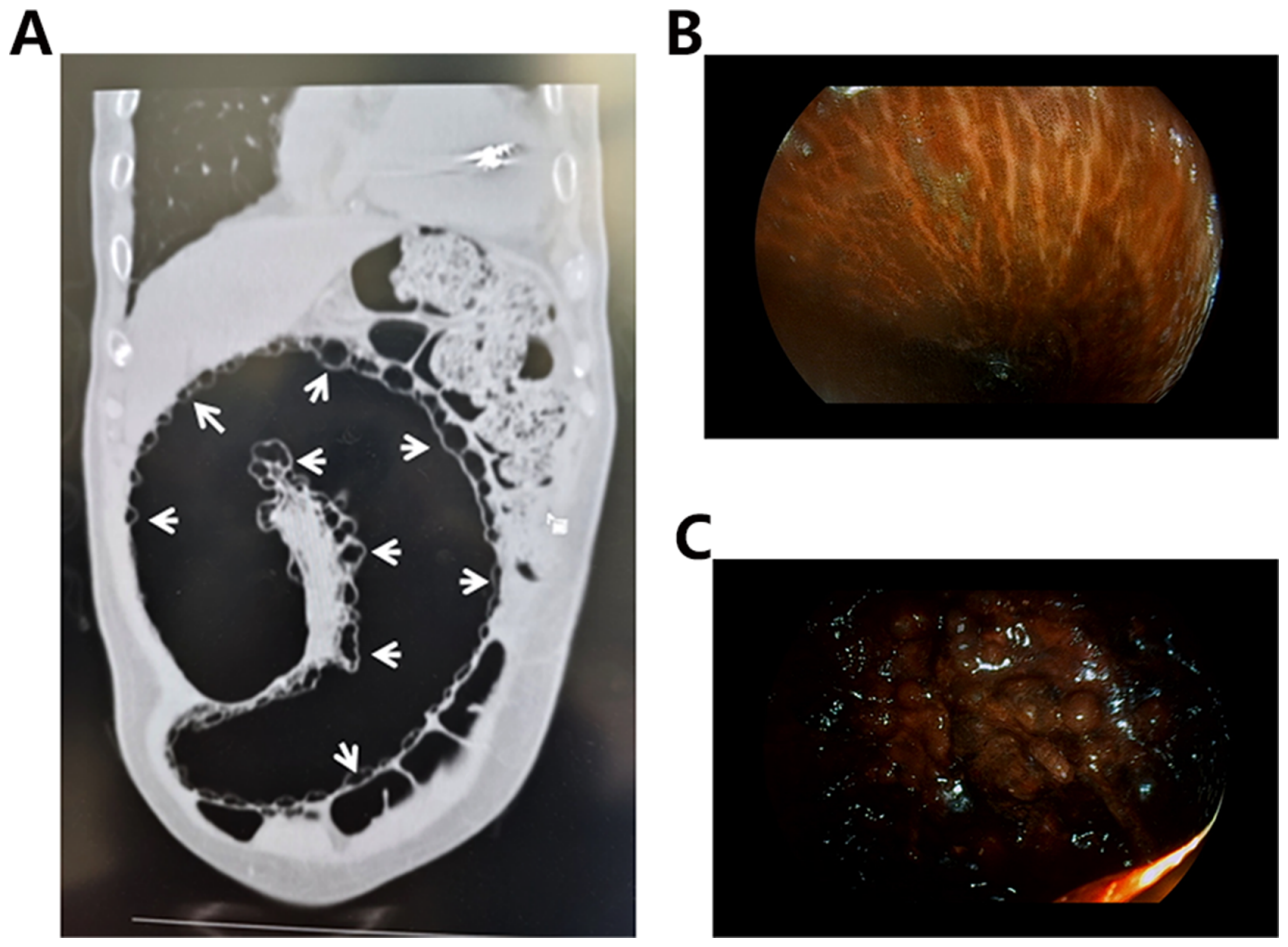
(A) Abdominal CT shows a number of grape-like or beaded low-density cystic light transmission areas in the bowel wall (white arrows). (B) Endoscopic images of the MC show brown or black pigmentation of the intestinal mucosa, presenting a crocodile-like appearance. (C) Endoscopy shows darkening of the intestinal mucosa with ischemic and necrotic changes.
